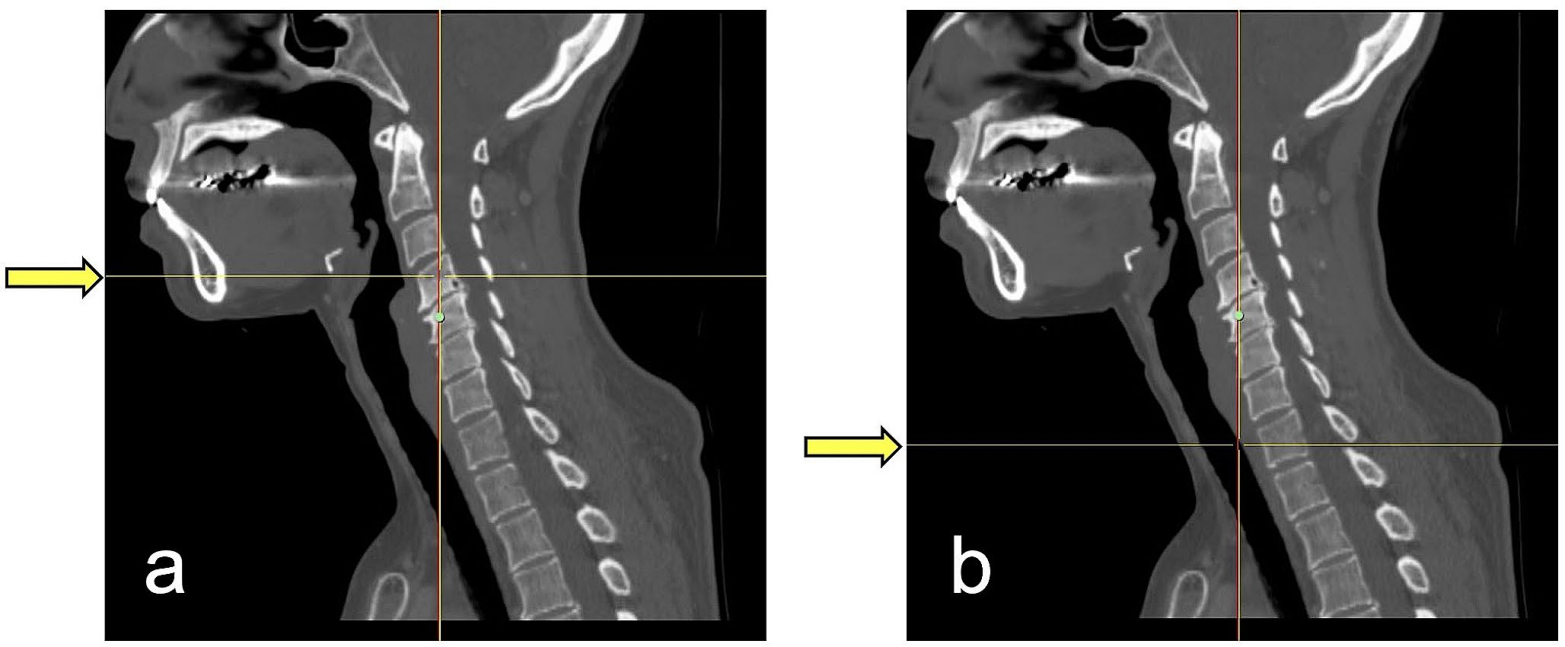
Examples of measurement of the vertebral level corresponding to the slice of CE-CT images of anatomical structures. (a) Vertebral level of central inferior border of hyoid bone (HB). The location of HB corresponded to the fourth cervical vertebra in this case. (b) Vertebral level of inferior border of cricoid cartilage (CC). The location of CC corresponded to the first thoracic vertebra in this case
